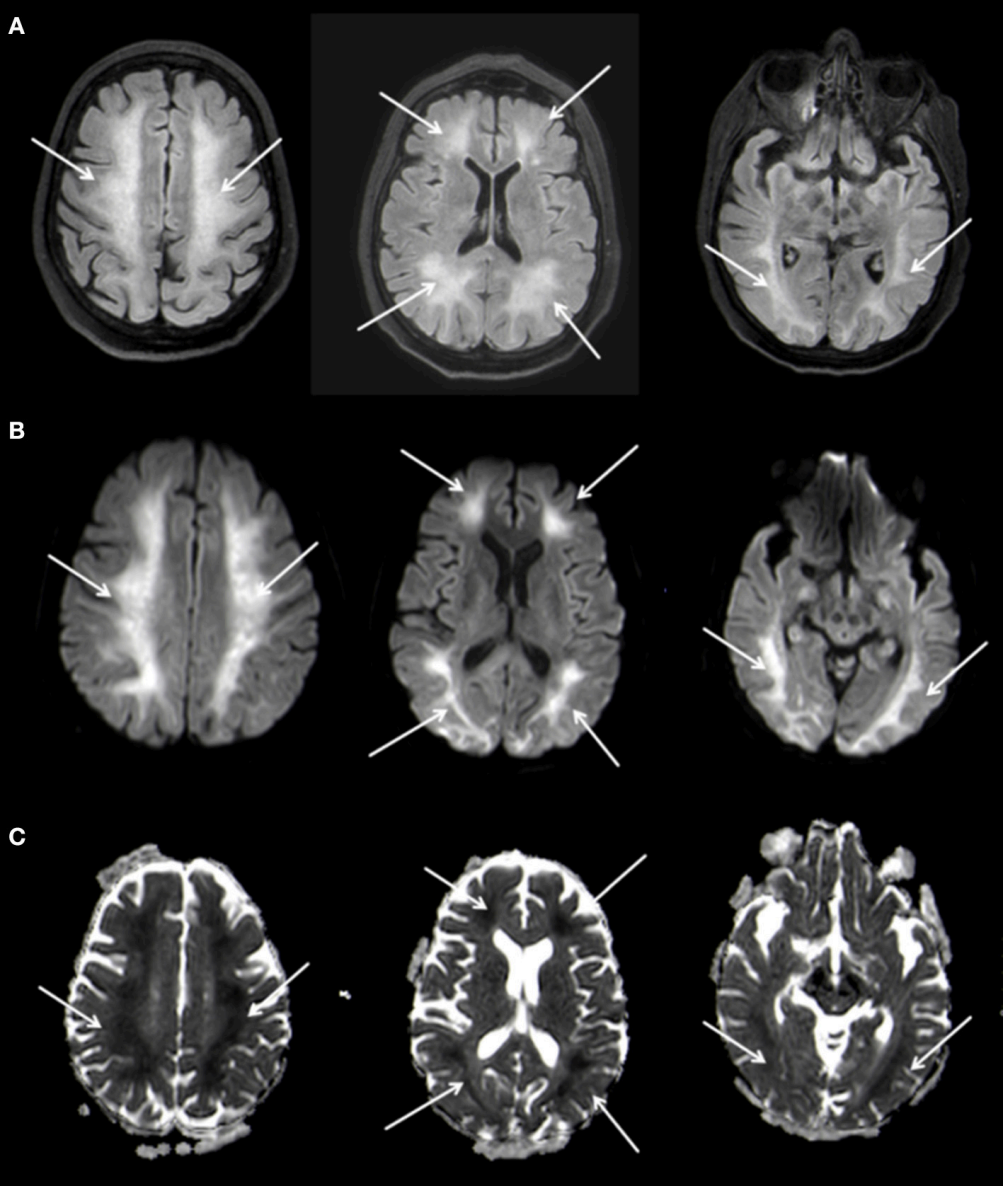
Planar T2w hyperintense signal changes of the entire white matter (A) with diffusion restriction (B,C) 3 T Philips Ingenia, (A) FLAIR, (B) b1000 Figure, (C) ADC Map.
